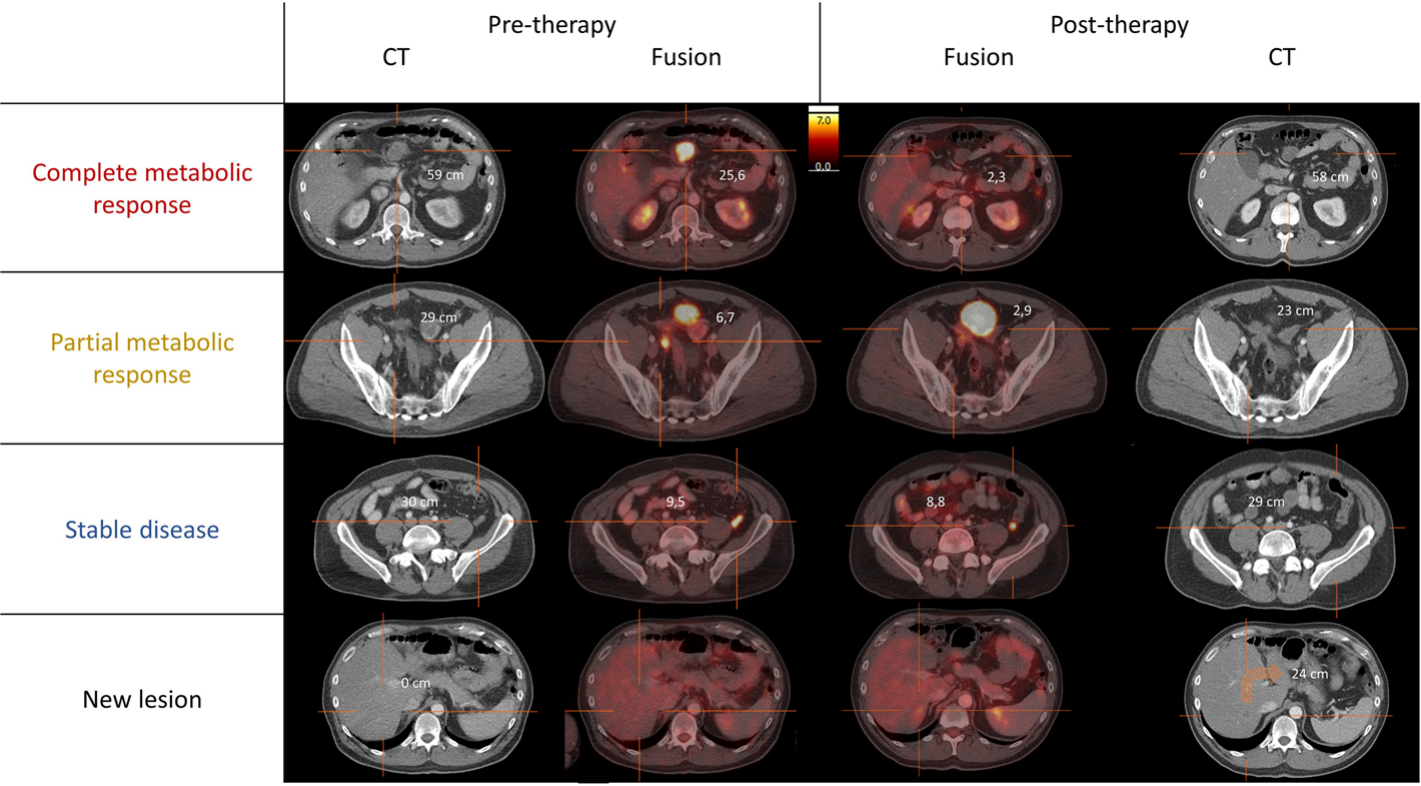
Pre- and post-therapy fused PET/CT and CT images of the different lesions
